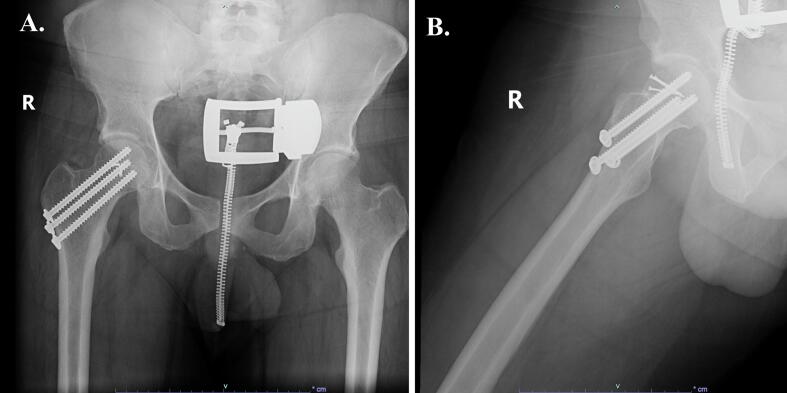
Radiographs of the right hip demonstrating (A) AP and (B) lateral views of the right hip at the final follow-up visit, ten months after the injury.
